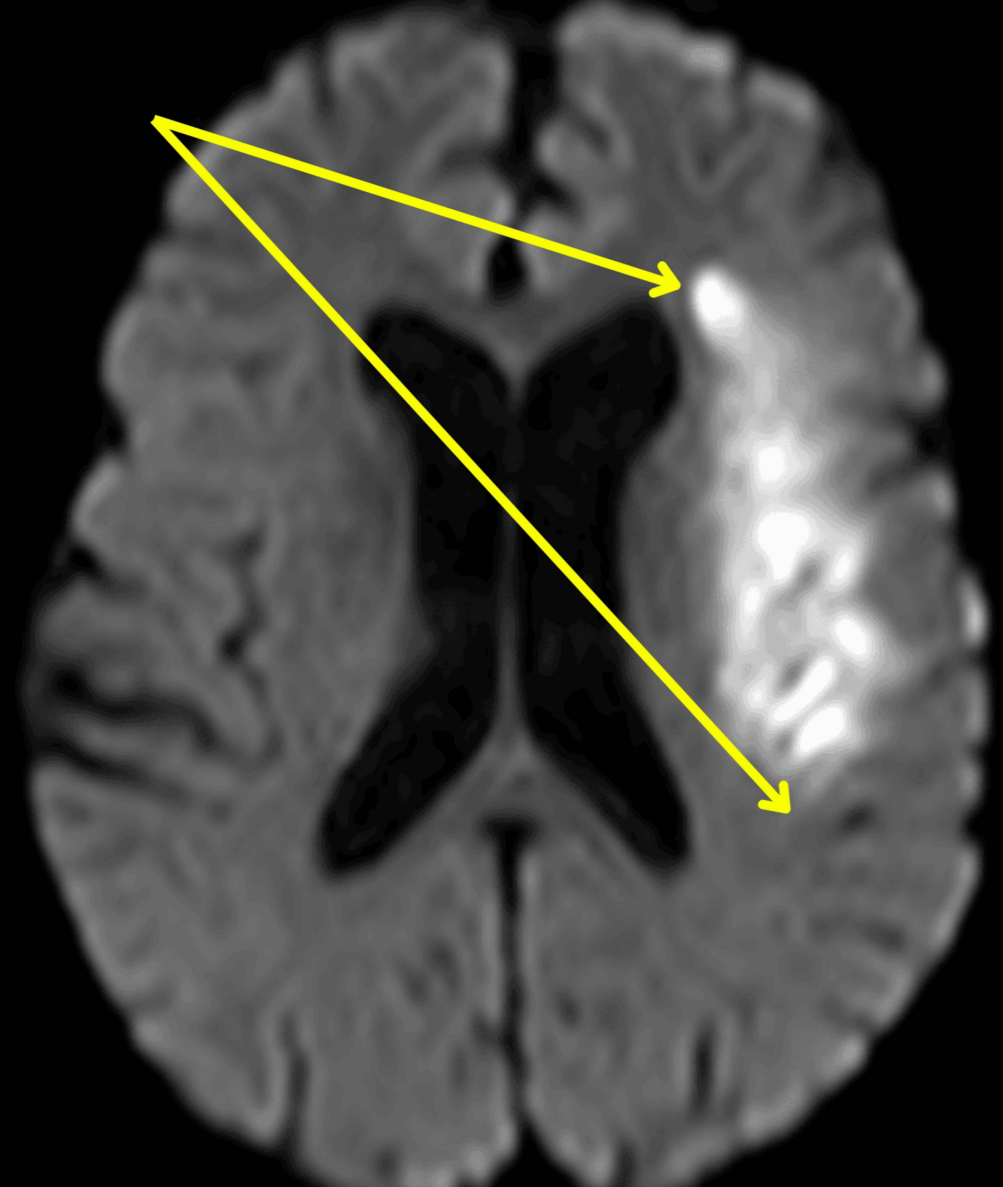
Axial section of MRI head showing acute non-hemorrhagic infarct in the left middle cerebral artery (MCA) territory Axial section of MRI head showing an acute non-haemorrhagic infarct in the left MCA territory. The infarction is indicated by the arrow.
